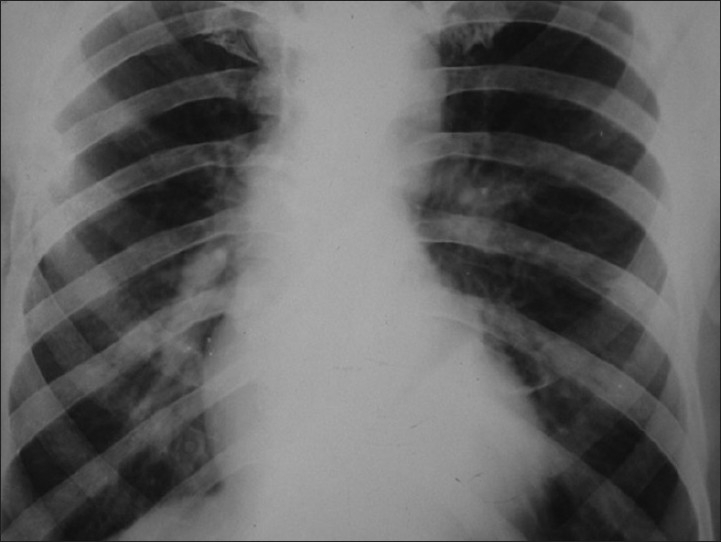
Chest radiograph showing right hilar prominence
